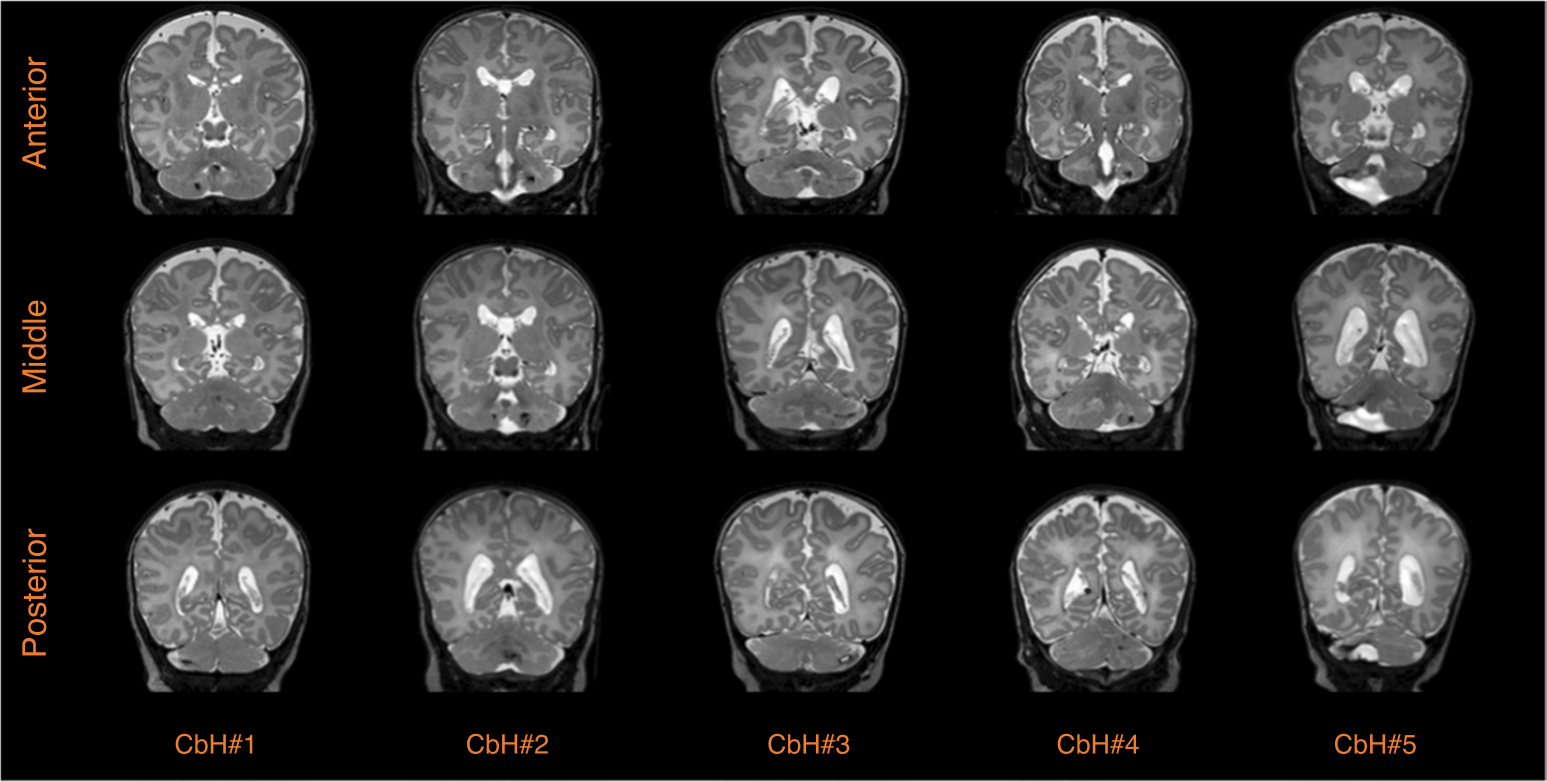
Coronal T2-weighted images on the size and location of the hemorrhages in the five infants with CbH at term equivalent age. To capture the three-dimensionality of the hemorrhages we provided a frame of the anterior, the middle and the posterior part of the hemorrhage.
